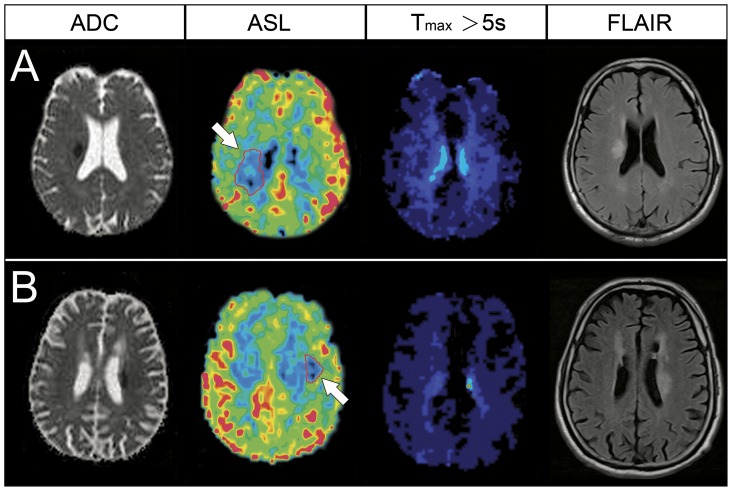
ADC, ASL perfusion (CBF), DSC perfusion (T_max_ >5 s), and follow-up FLAIR imaging of the representative patients. (A) A 76-year-old man underwent MRI at 5.0 hours after stroke onset. An acute infarct was noted in the right centrum semiovale in the ADC and follow-up FLAIR images. A perfusion defect was observed in the ASL map (arrow) but not in the T_max_ map. (B) A 60-year-old man underwent MRI at 22.6 hours after stroke onset. The ADC image showed an acute infarct in the left centrum semiovale with a corresponding perfusion defect in the ASL map (arrow), but not in the T_max_ map.
